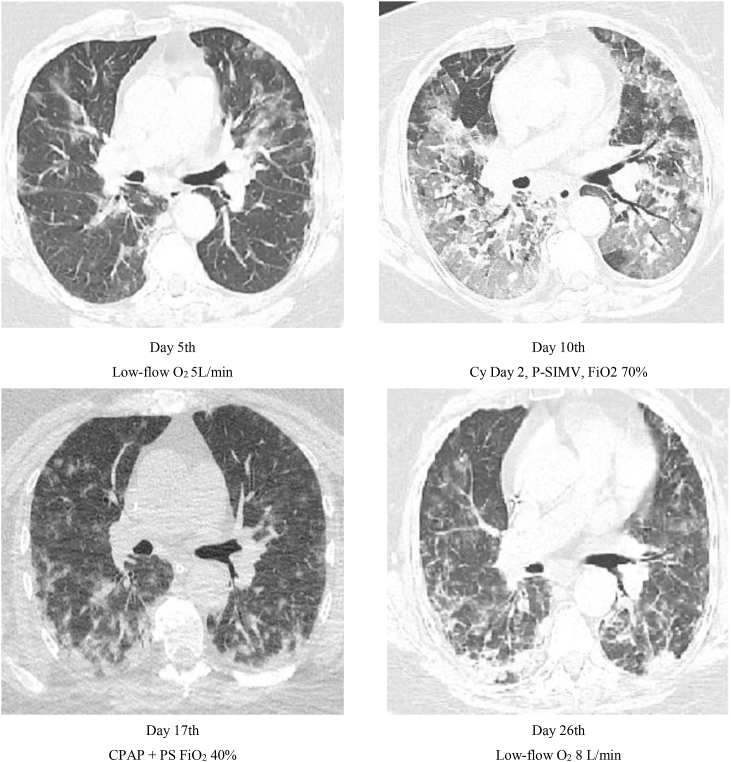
Lung CT scans of Patient 2.
